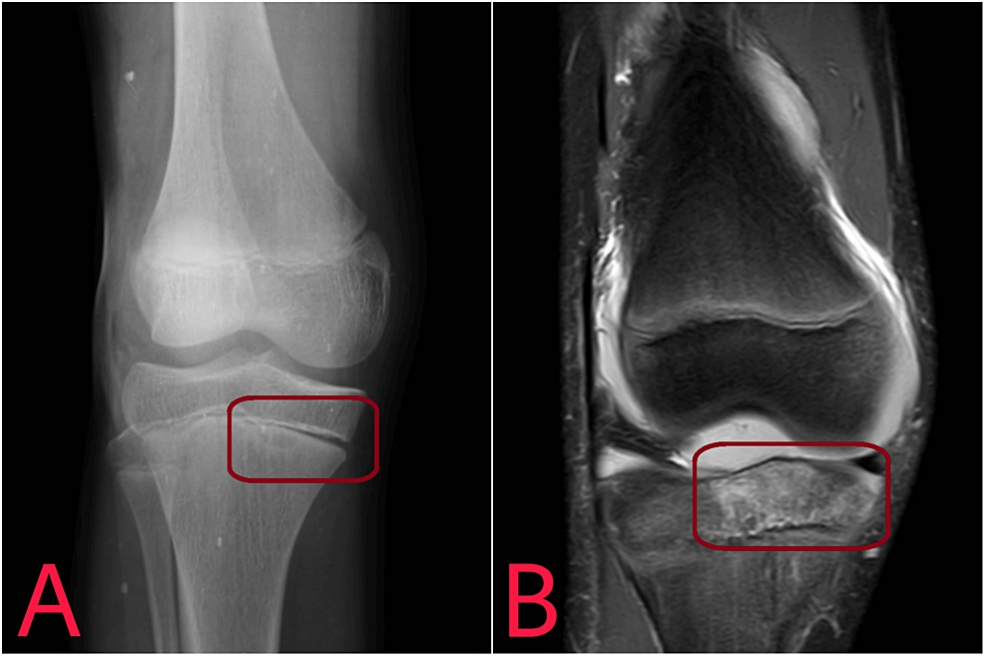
An X-ray (A) and MRI (B) showing Salter-Harris Classification Type I, i.e., epiphyseal slip and separation through the physis of proximal tibia medially.
